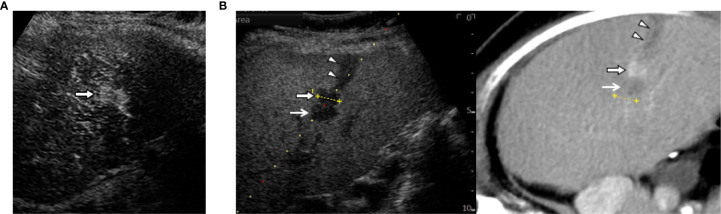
The combination of contrast-enhanced US (CEUS) and fusion imaging. (A) CEUS with Sonazoid shows arterial enhancement of locally progressed hepatocellular carcinoma (HCC) (arrow). (B) The combination of Kupffer image on contrast-enhanced US (CEUS) and fused CT image demonstrates viable HCC (arrow) sandwiched between an ablated tract (arrow heads) and a necrotic tumor (open arrows).
